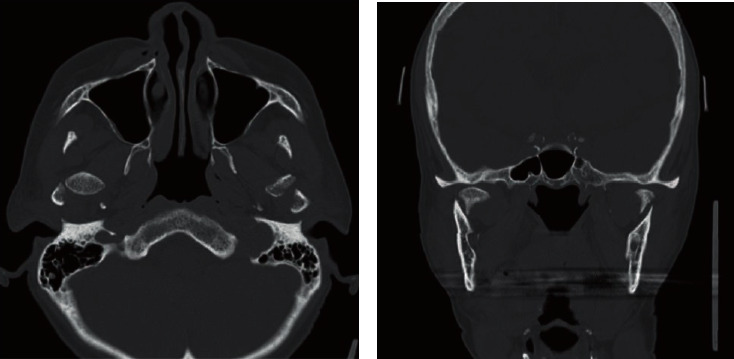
CT findings: (a) sagittal reconstruction of CT images and (b) coronal reconstruction of CT images. The bilateral condylar fractures were observed.
